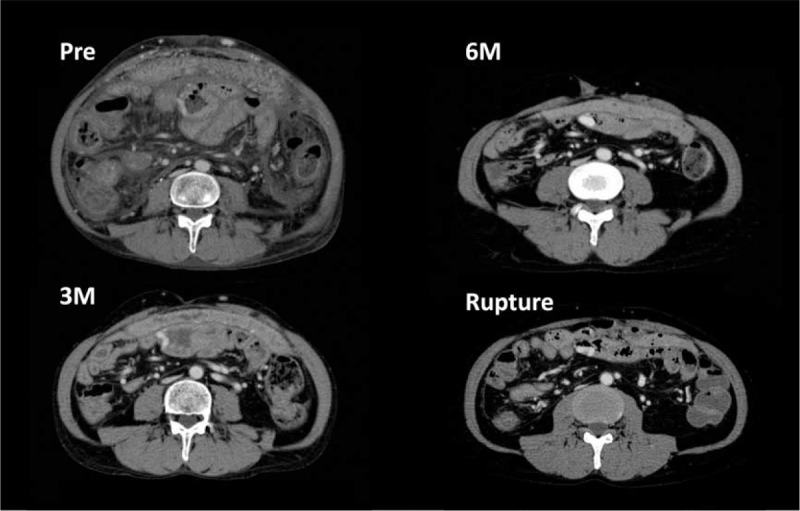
Computed tomography images of the jejunal varices taken at pretransplant, 3 months, 6 months, and readmission.
